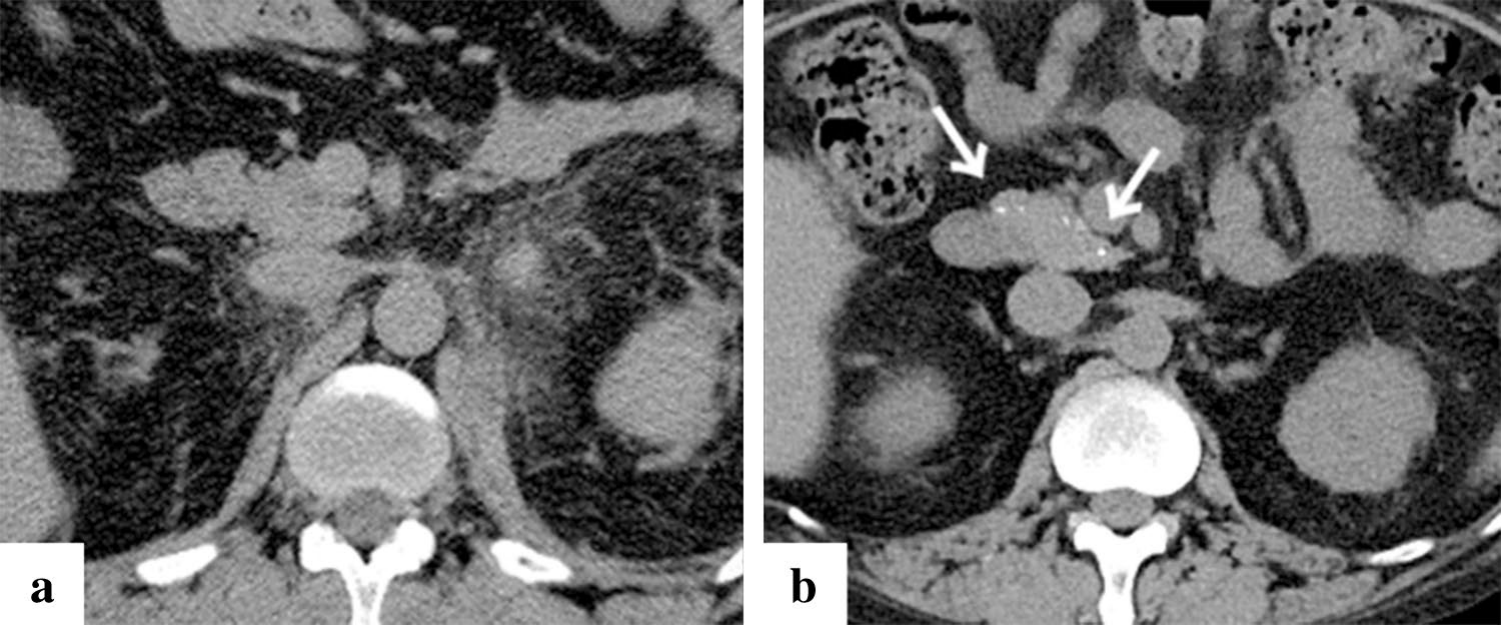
Representative case of pancreatic calcification in a 43-year-old patient (case no. 8). Computed tomography (CT) imaging on day 11 since the onset of symptoms (a) and after one month of the admission (b). CT imaging on admission showed no evidence of acute inflammation in the pancreas (a), and CT imaging one month after admission revealed calcification of the pancreas (b, white arrow). In addition, CT imaging on admission showed bilateral retroperitoneal oedema (a)
